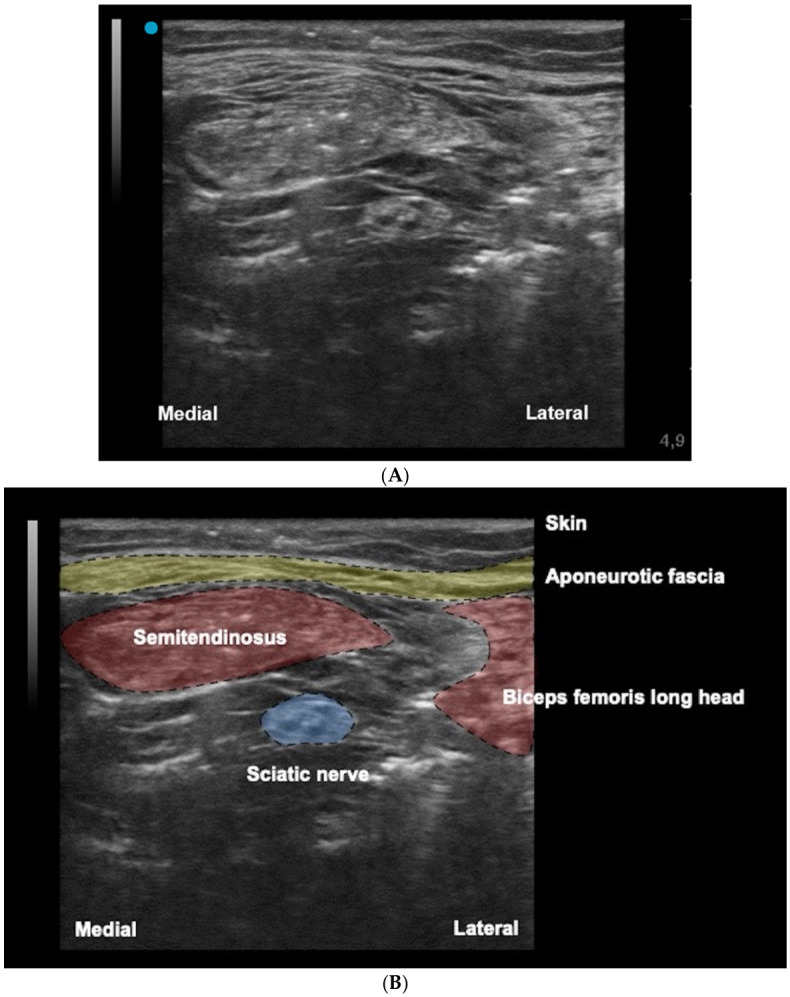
Short-axis ultrasound image of the sciatic nerve at the injection level. (A) Unannotated ultrasound image. (B) Annotated image corresponding to panel (A). The skin, aponeurotic fascia, semitendinosus muscle, biceps femoris long head, and sciatic nerve are labeled. Medial and lateral orientations are also indicated.
